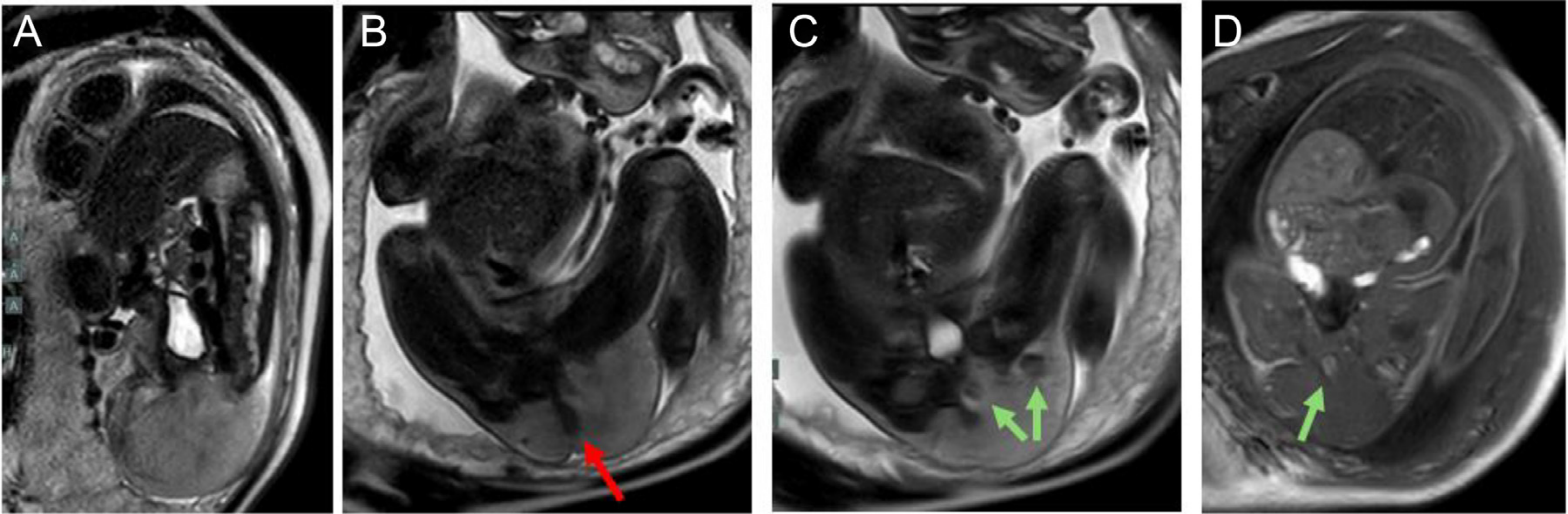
Fetal MRI performed at 35 weeks and 4 days gestation. Sagittal T2 weighted image (A) reveals a large mass centered on the pelvis but no extension into the spinal canal. Coronal T2 weighted images (B, C) show the displacement of the rectum (red arrow) with areas of hemorrhage (green arrows). The mass is hyperintense to skeletal muscle. Coronal T1 image (D) shows that the mass is isointense to skeletal muscle. Foci of hemorrhage are again noted (green arrow).
